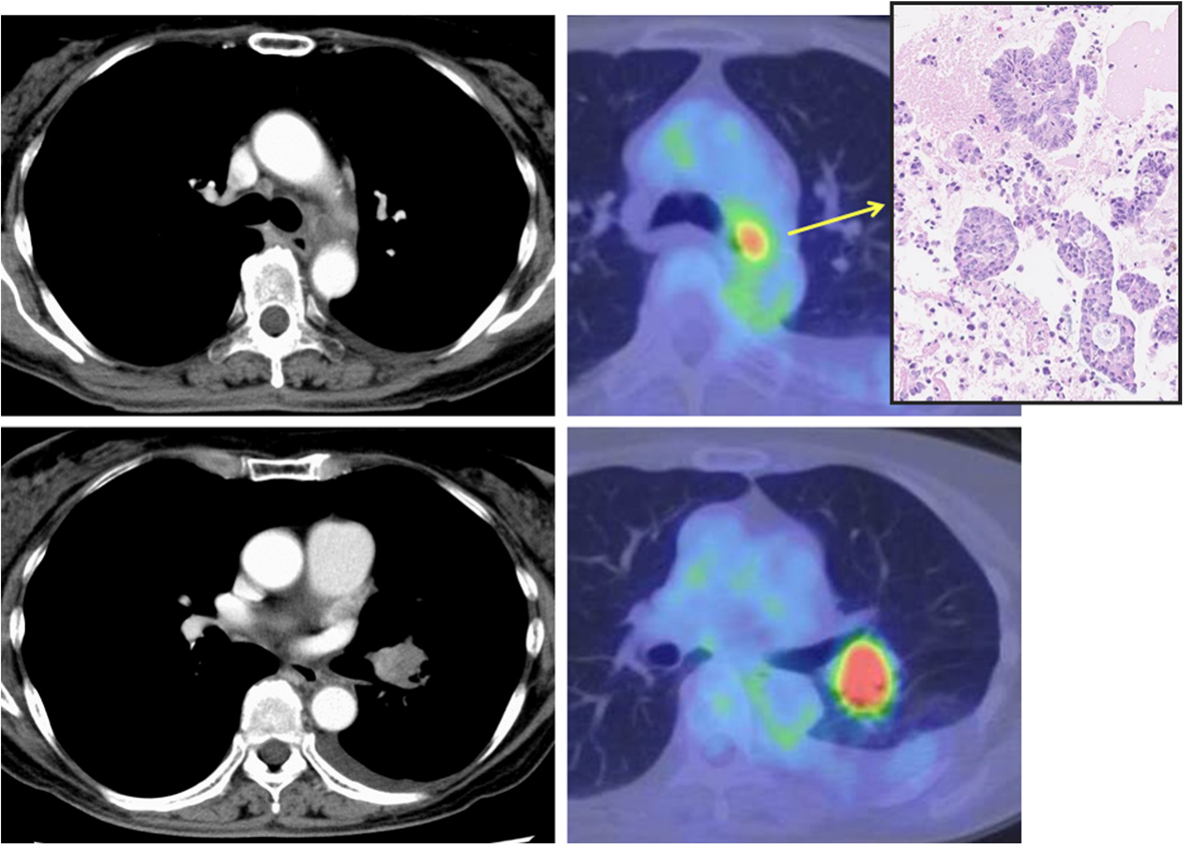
Computed tomography and positron emission tomography before the treatment
